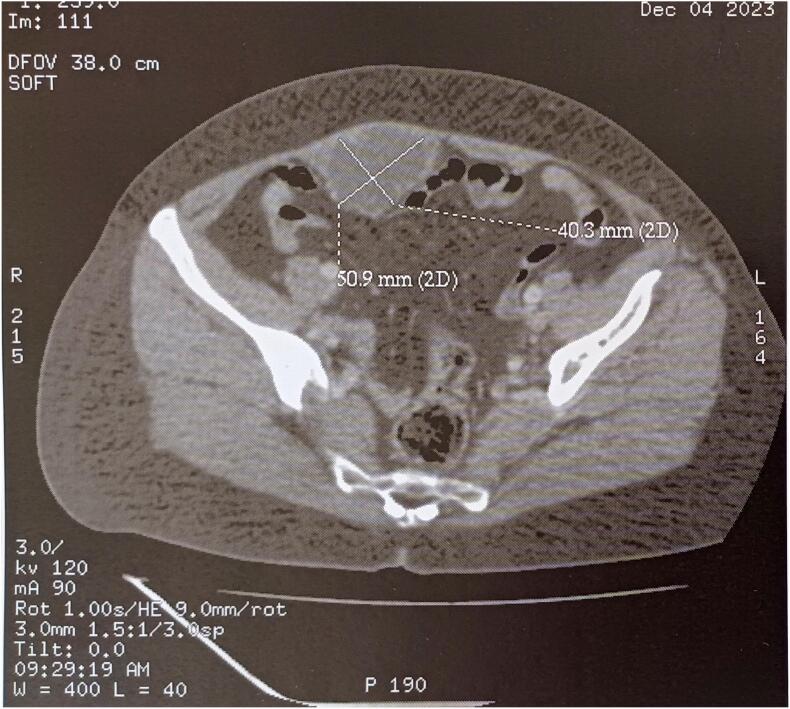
Sagittal CT scan depicted a solid-cystic parietal formation within the rectus abdominis muscle, situated below the umbilicus, featuring a budding tissue portion measuring 50 × 40 × 70 mm.
